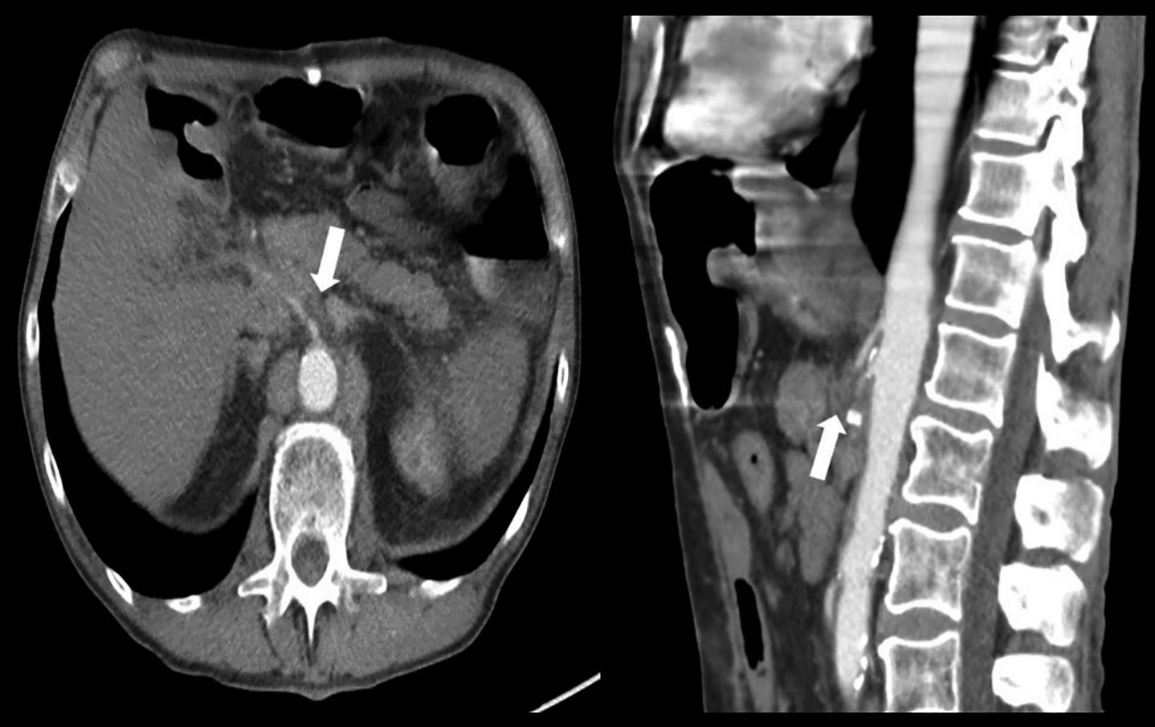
Computed tomography (CT) abdomen and pelvis with intravenous contrast (axial and sagittal planes) showing an ill‐defined soft tissue mass surrounding the celiac artery and superior mesenteric artery with dense calcification concerning for NET recurrence (arrow).
